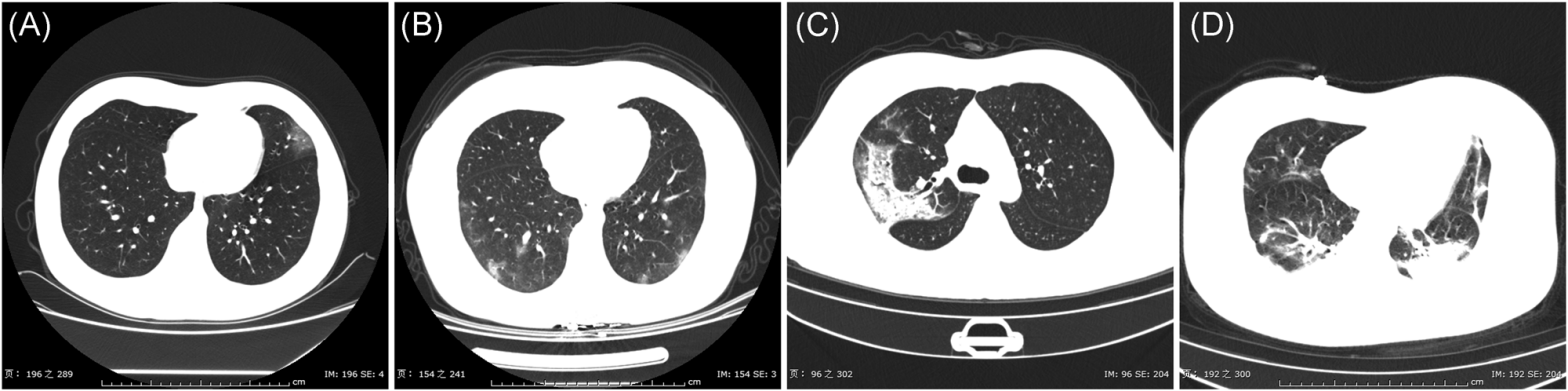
Chest CT Manifestations of COVID‐19. A, Single GGO; B, diffuse GGO; C, consolidation; D, both GGO and consolidation. COVID‐19, coronavirus disease 2019; CT, computed tomography; GGO, ground‐glass opacities
